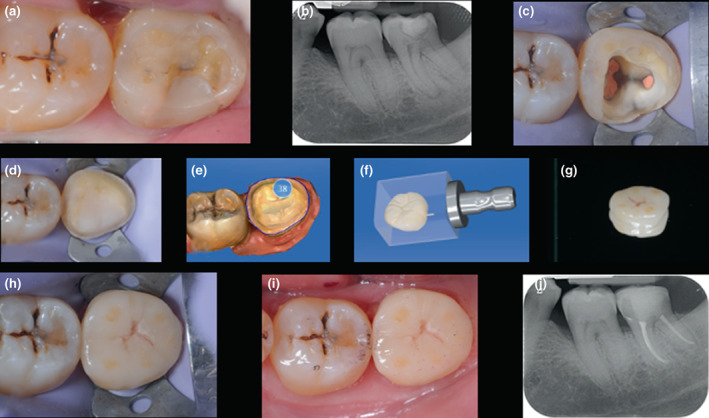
Lithium disilicate onlay (IPS e.max CAD) placement following root canal treatment of symptomatic 38 (a, b) preoperative occlusal view and long‐cone periapical radiograph (c) completed root canal treatment and dentine surface preparation (d) completed core and onlay preparation (e–g) the onlay is fabricated using computer‐assisted design and manufacturing (CAD‐CAM) (h, i) finished restoration with postoperative occlusal view and follow‐up periapical radiograph (j).
